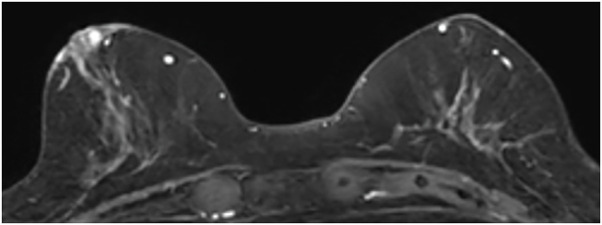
Findings on contrast-enhanced MRI. A 2.5-mm nodule was noted slightly medial to the right nipple. This nodule demonstrated a fast-persistent pattern on contrast enhancement, suggesting possible intraepithelial spread of Paget’s disease into the nipple. Periductal skin thickening was also interpreted as tumor infiltration. Within the breast, small nodules with contrast enhancement were noted within a 2-cm range from the nipple, suggesting ductal spread. No obvious lymph node enlargement was observed.
